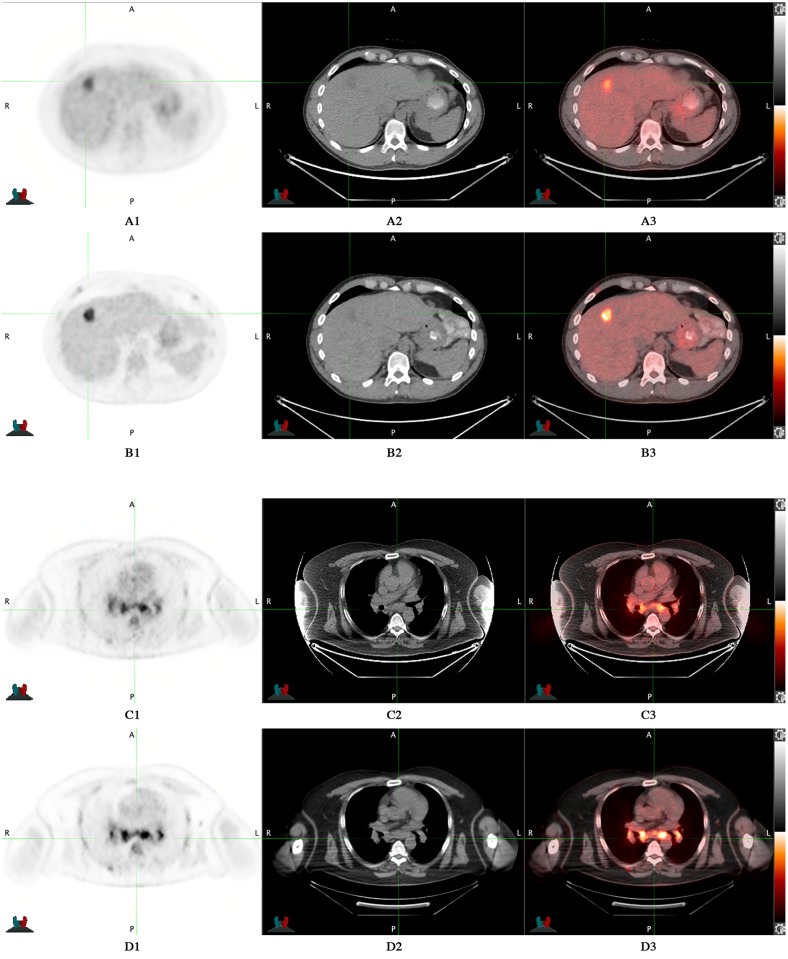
D690 PET/CT vs DMI PET/CT at different time delay between scans. A1) D690 PET; A2) D690 CT; A3) D690 fused image; B1) DMI PET; B2) DMI CT; B3) DMI fused image. Time delay between scans was 18 minutes. C1) D690 PET; C2) D690 CT; C3) D690 fused image; D1) DMI PET; D2) DMI CT; D3) DMI fused image. Time delay between scans was 73.7 minutes.
